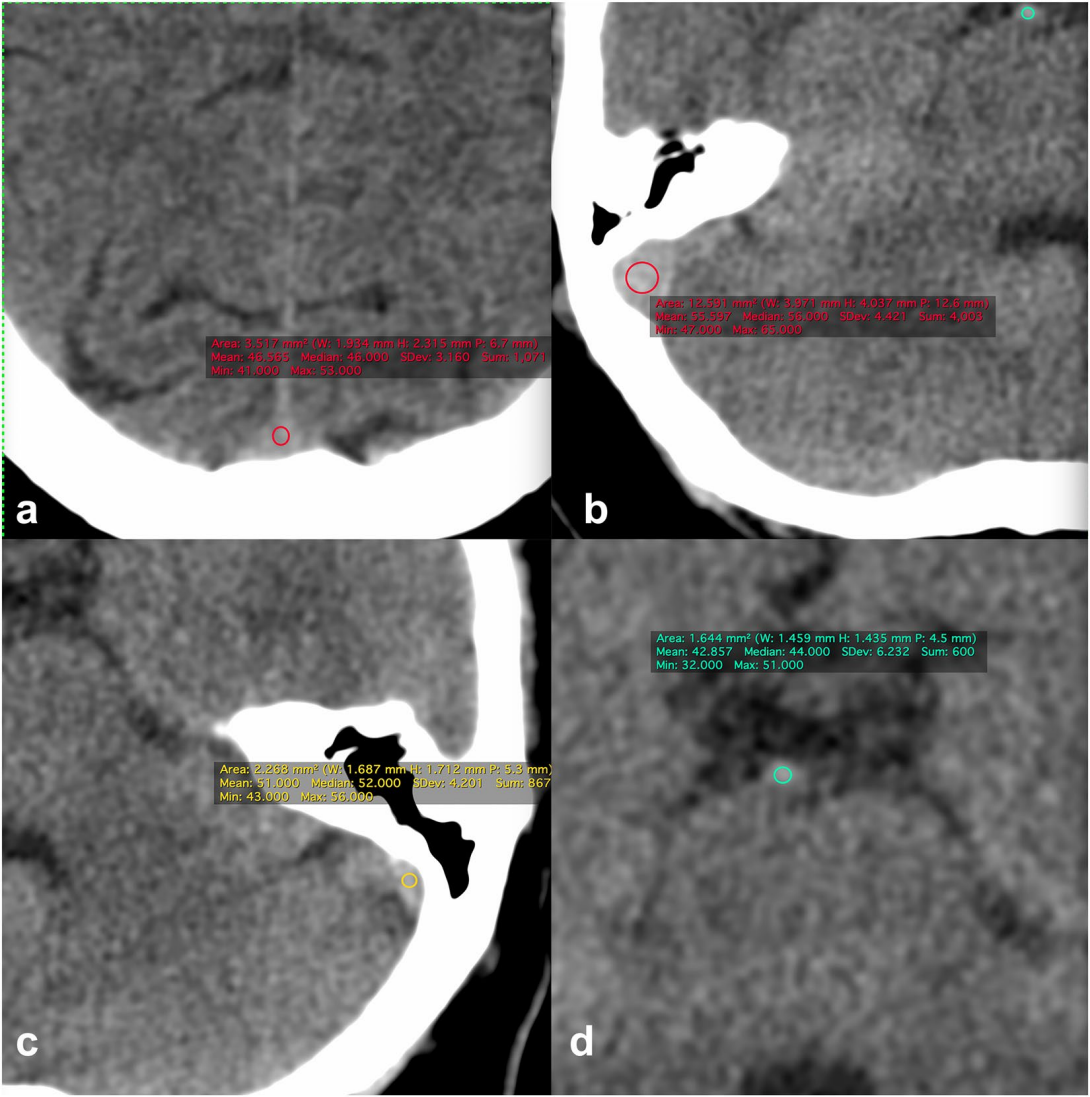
Zoomed in images of axial brain CT scans shows blood density measurement in the superior sagittal (a), right sigmoid (b), left sigmoid (c) sinuses and basilar artery (d). All images are first zoomed in, and a ROI is placed in the center of vascular structure and is not in contact with the vessel wall.
